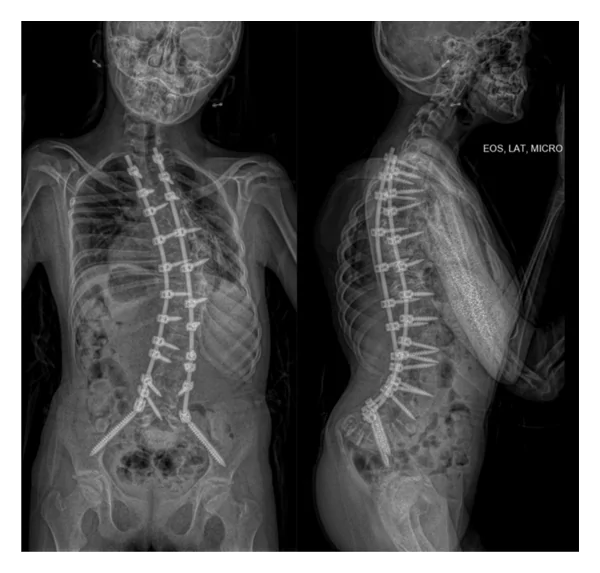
Postoperative radiographs, coronal and sagittal views showing correction in spinal deformity.
